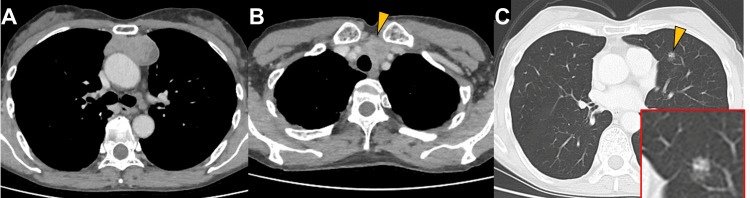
CT of the mediastinal tumor, neck mass, and lung nodule CT showing (A) a 60-mm anterior mediastinal mass in contact with the pericardium; (B) a swollen lymph node in the neck (arrowhead), and (C) multiple small nodules in bilateral lungs (arrowhead).
